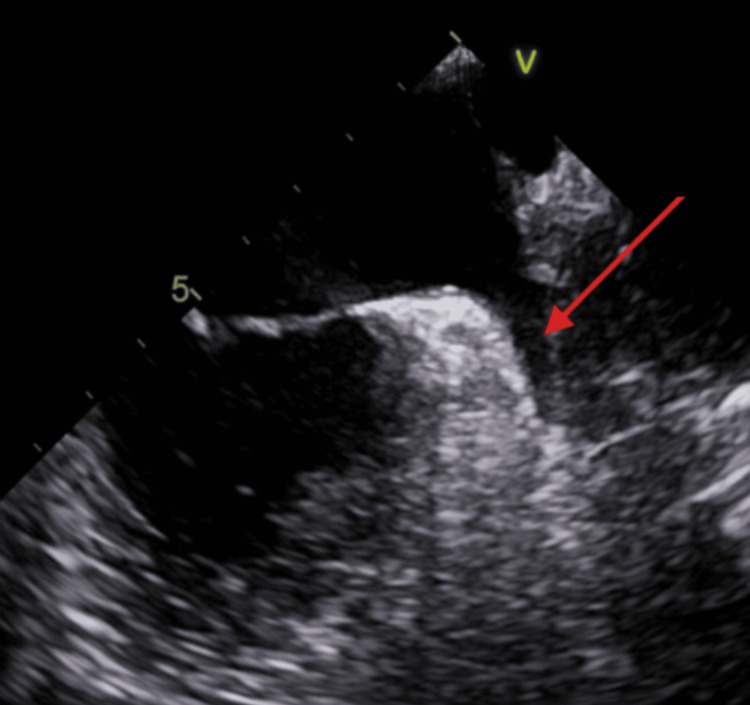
Transesophageal Echocardiographic View of the Left Atrial Appendage TEE image of the left atrial appendage without thrombus, with the red arrow indicating the appendage. TEE: transesophageal echocardiography
